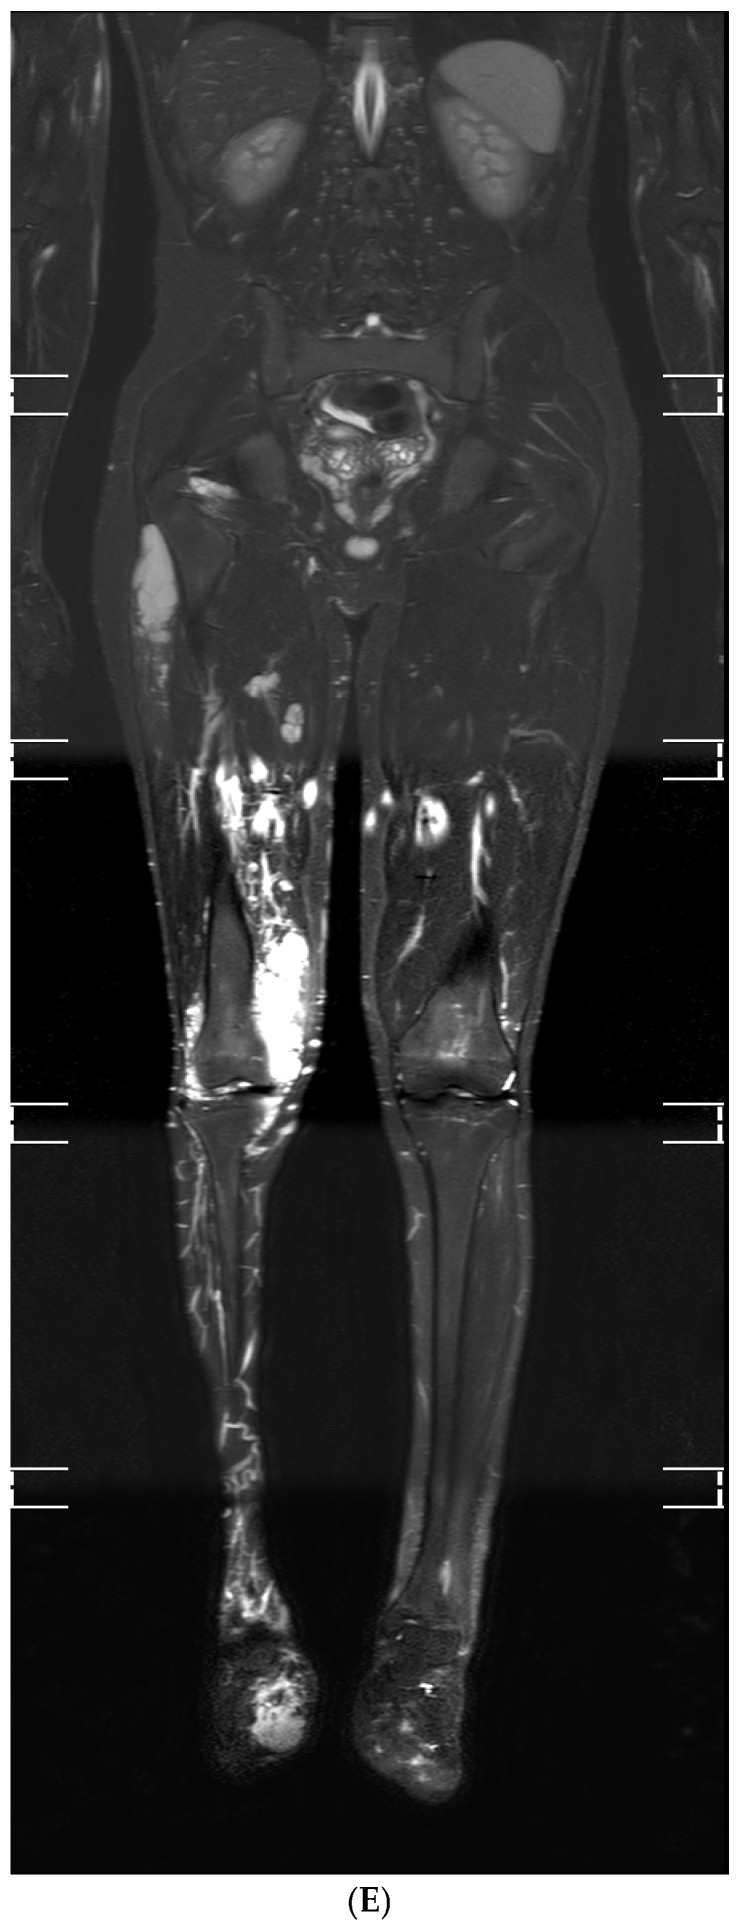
(A) PD-weighted image with fat saturation in axial plane demonstrating intra-articular venous malformation encompassing distal femoral epiphysis, (B) PD-weighted image with fat saturation in sagittal plane demonstrating intra-articular venous malformation involving infrapatellar (Hoffa’s) fat pad, (C) PD-weighted image with fat saturation in coronal plane demonstrating intra-articular venous malformation involving knee joint, (D) T2-weighted image with fat saturation comparing venous malformation involving right knee joint with normal tissues of left lower extremity, (E) T2-weighted STIR image showing venous malformation involving entire right lower extremity.
